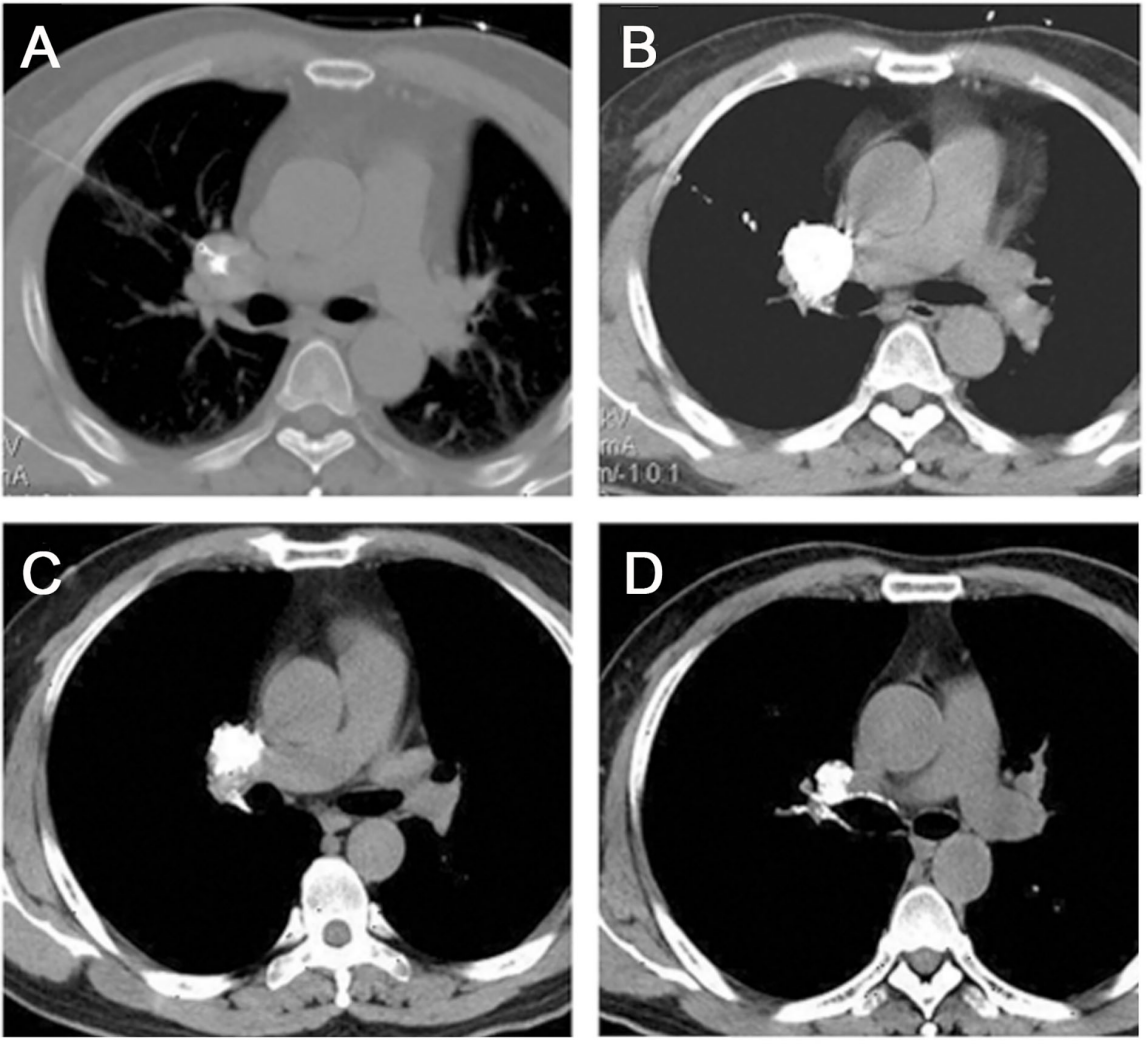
Chemical ablation of a lymphatic node metastasis in the right pulmonary hilar region. (A) Transverse CT before chemical ablation showed a tumor at the lung hilum. The needle tip was located in the center of the target LN. (B) The image obtained after a single injection of the ethanol-ethiodol-drugs mixture showed good LN permeation. (C-D) At 6 and 12 months after ablation, the tumor size gradually decreased. (Liu SR et al., Technol Cancer Res Treat, 2013).
